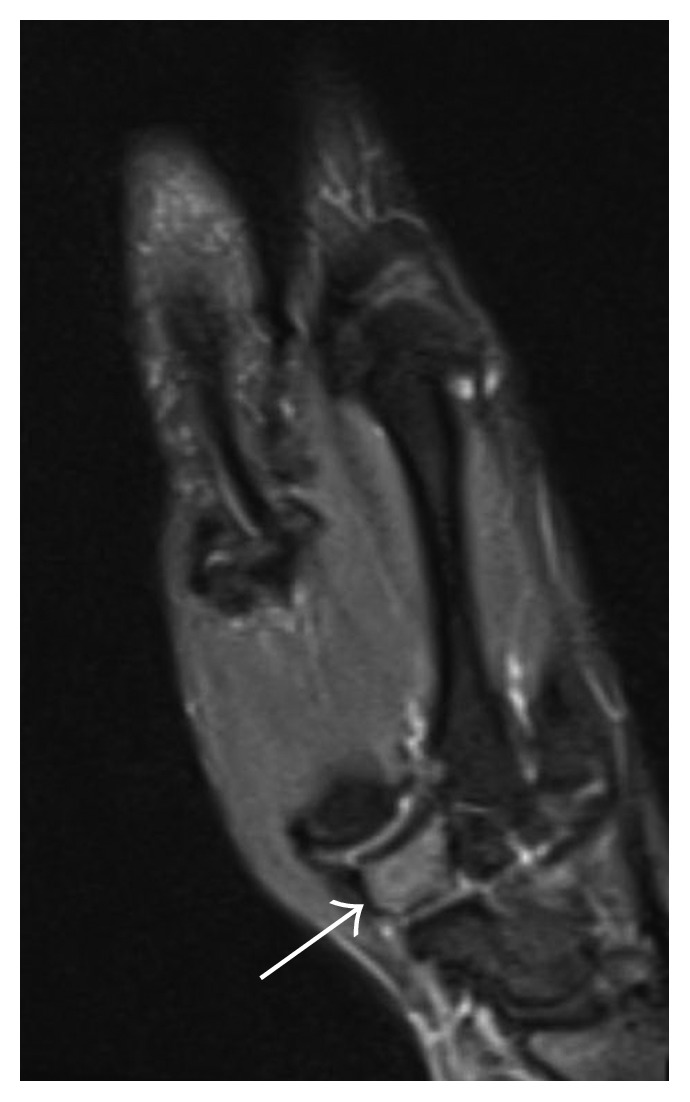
Magnetic resonance imaging demonstrating circular hypointense lesion (arrow).
